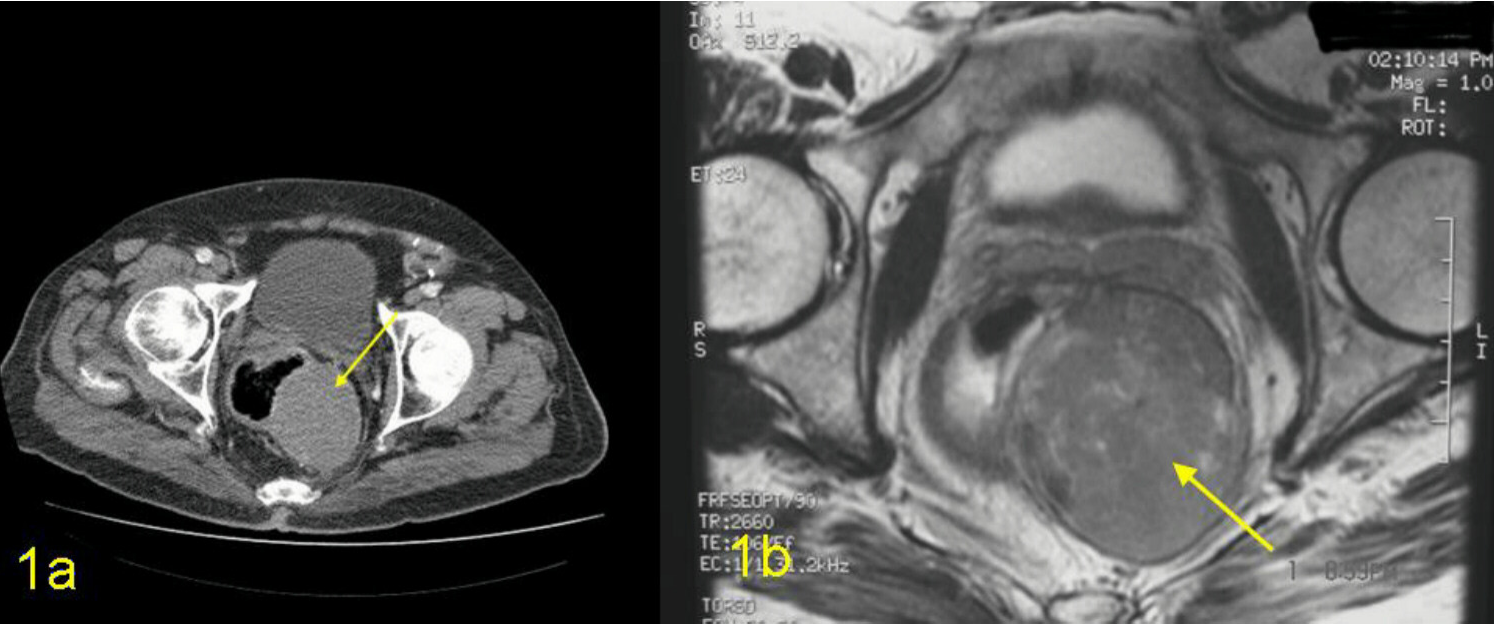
Imaging of the large bowel 1a) CT scan showing the large lesion in the posterior and left lateral wall of the rectum and 1b) MRI imaging: the distal rectal wall lesion (GANT) is shown to be intramural and free from adjoining structures.
